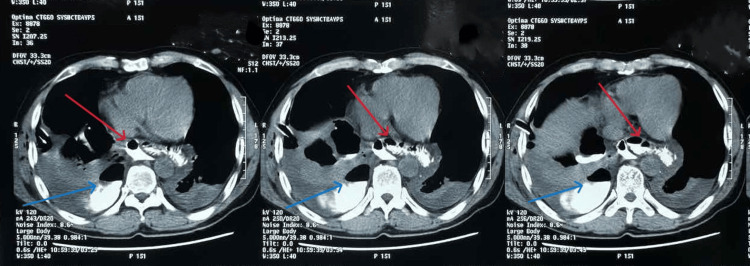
CT showing contrast dye in the esophagus leaking into the mediastinum and right pleura (red arrow) – moderate right pleural effusion (blue arrow)
